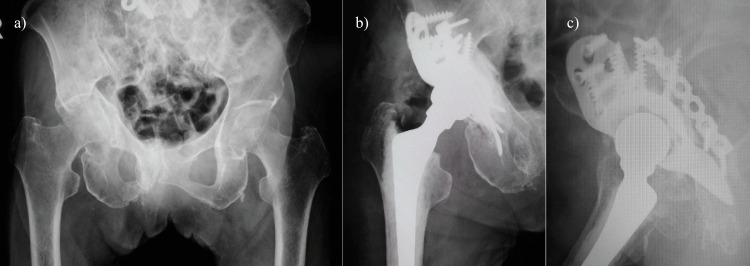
Radiographs of case 1: a 78-year-old female patient a) Preoperative radiograph, b) Postoperative radiograph (anterior-posterior view), and c) Postoperative radiograph (lateral view)
